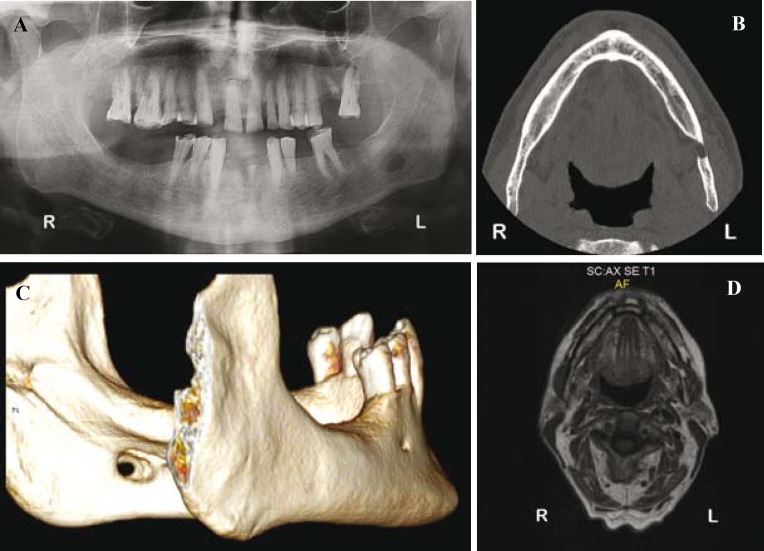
A) Panoramic radiography showing a well-defined, oval, radiolucent lesion under the left mandibular canal. B) Axial section of the CT scan of the mandible showing involvement of both lingual and buccal plates. C) 3-D reconstruction of the CT showing tunnel-type lesion. D) Coronal T1-weighted MR image. The soft tissue included in the defect is contiguous to the adjacent submandibular gland.
